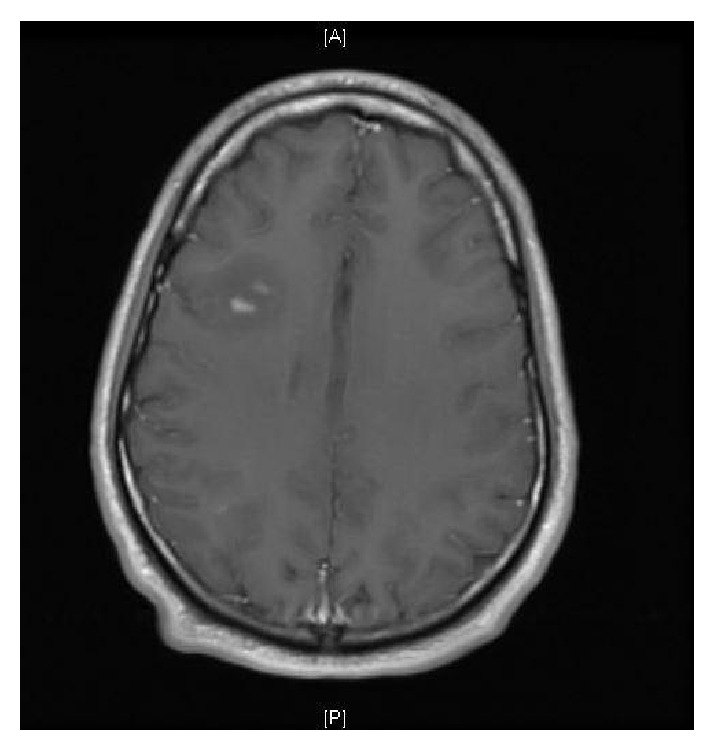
A T1-weighted postgadolinium magnetic resonance image demonstrating two nodular foci of enhancement with surrounding vasogenic edema.
